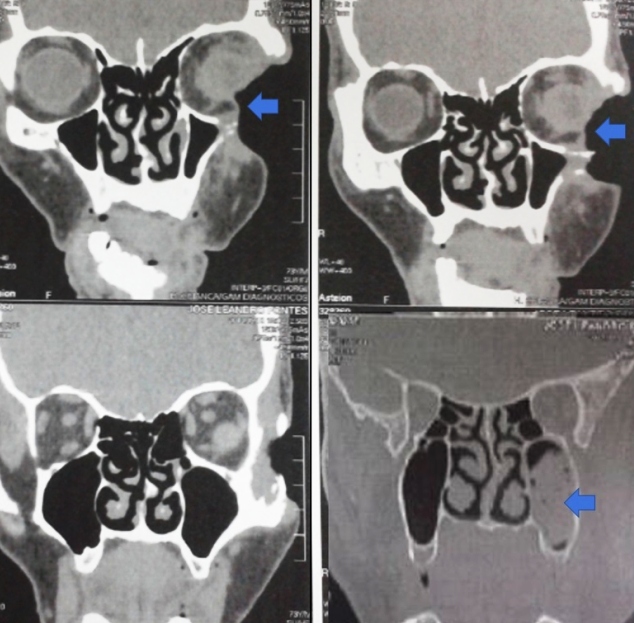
Destruction of the left orbit and left zygomatic bone. Left maxillary sinus filling (skull computed tomography).
